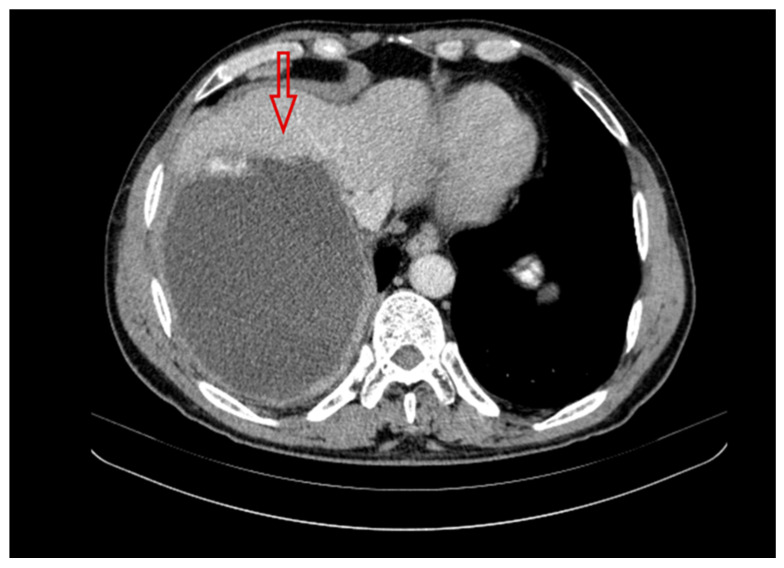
The computed tomography performed in the third year of treatment of a patient with AE. In the right lobe of the liver (red arrow), a parasitic lesion with a dominant fluid component is visible. The contours of the lesion are irregular and contain calcifications. CT photo of a patient treated at the Division of Tropical Medicine and Parasitic Diseases in Gdynia (M.S.; K.S.).
